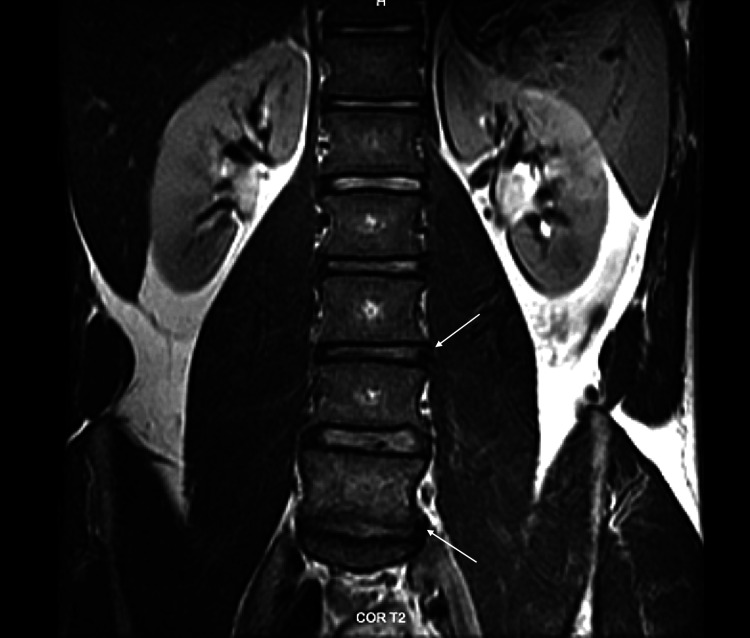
Coronal T2-weighted MRI of the lumbar spine demonstrating a lateral disc herniation compressing the left L4 nerve root and a central-to-right paracentral herniation impacting the right S1 nerve root (indicated by white arrows).
